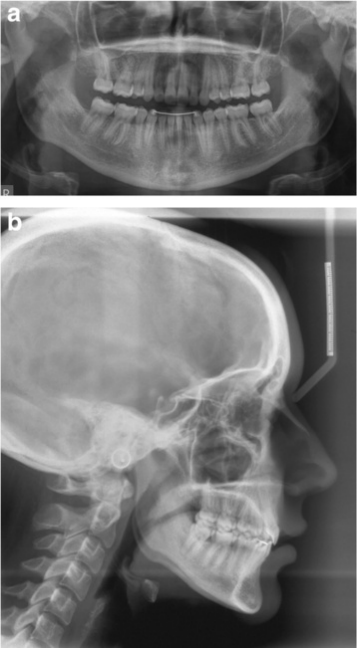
a Panoramic radiograph at the end of orthodontic treatment showing the 1st permanent molar in the position of the 2nd premolar. b Final cephalometric radiograph
